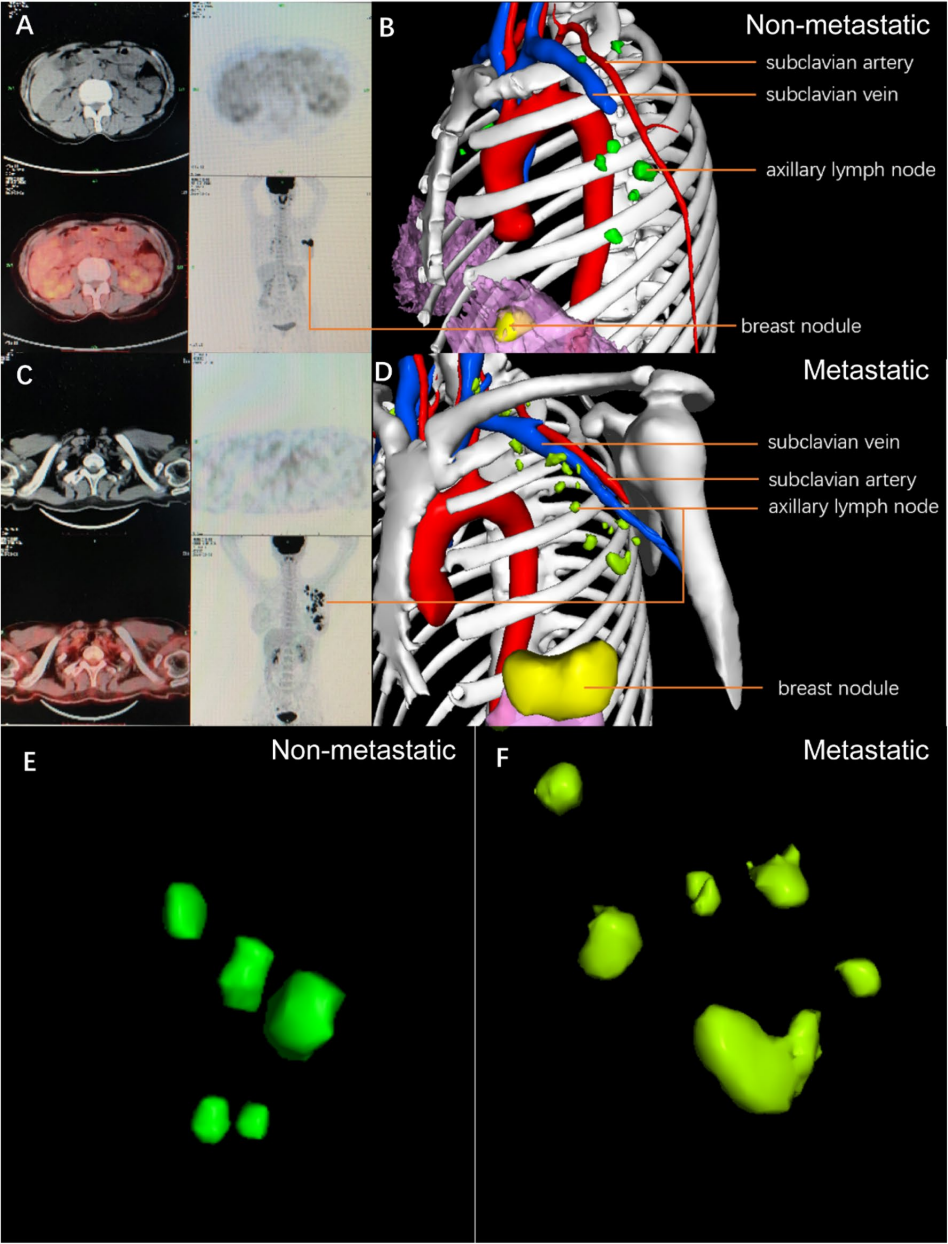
Comparison of PET-CT images with the 3D reconstruction system for the display of suspected metastatic lymph nodes. (A) and (C): Positron emission tomography/computed tomography images of non-metastatic (A) and metastatic patients (C); (B) and (D): 3D reconstruction images of non-metastatic (B) and metastatic patients (D); (E) and (F): 3D reconstruction local magnified images of non-metastatic (E) and metastatic patients (F).
